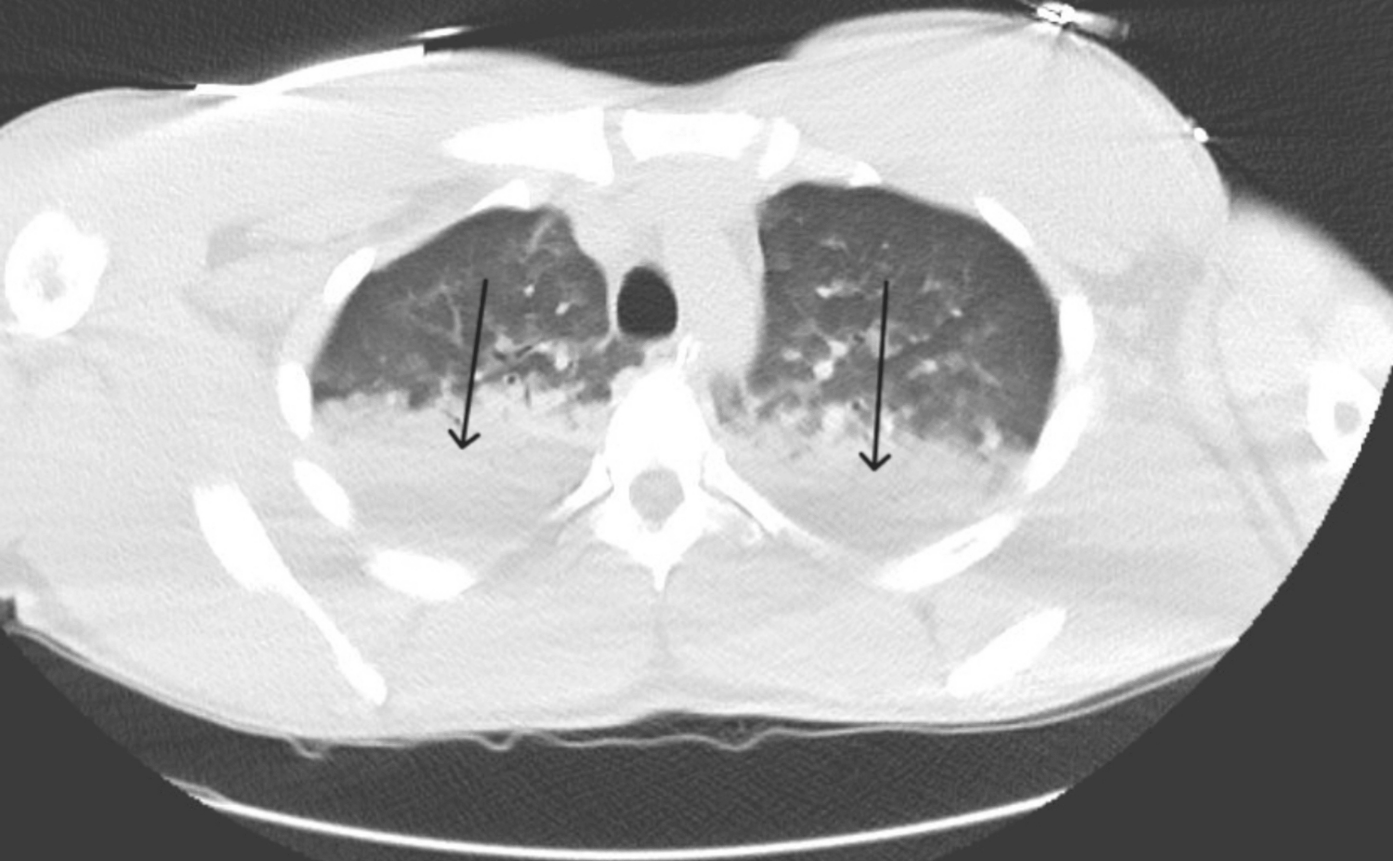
Chest CTA with arrows depicting pneumonitis. CTA, computed tomography angiography
